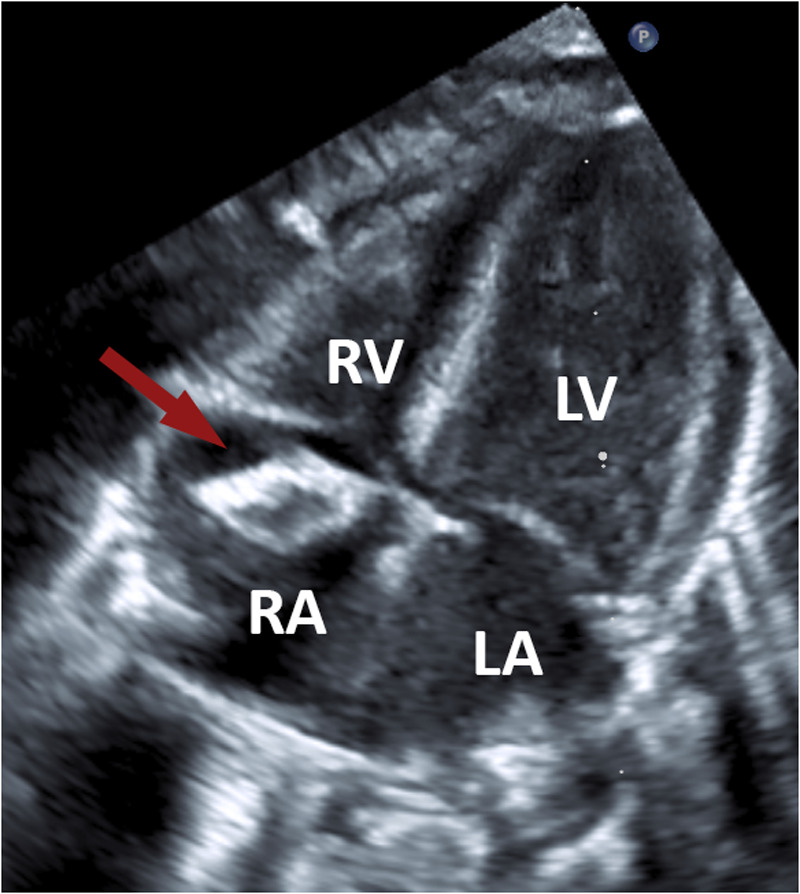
Transthoracic echocardiographic image demonstrating a mass (arrow) within the right atrium. RA, right atrium; LA, left atrium; RV, right ventricle; LV, left ventricle.
